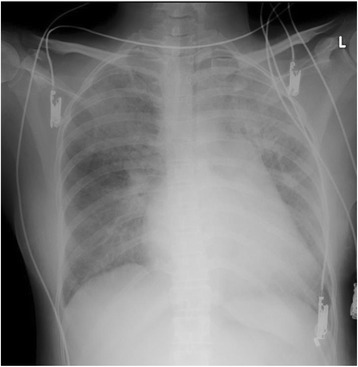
Chest X-ray showing the butterfly pattern of pulmonary edema
